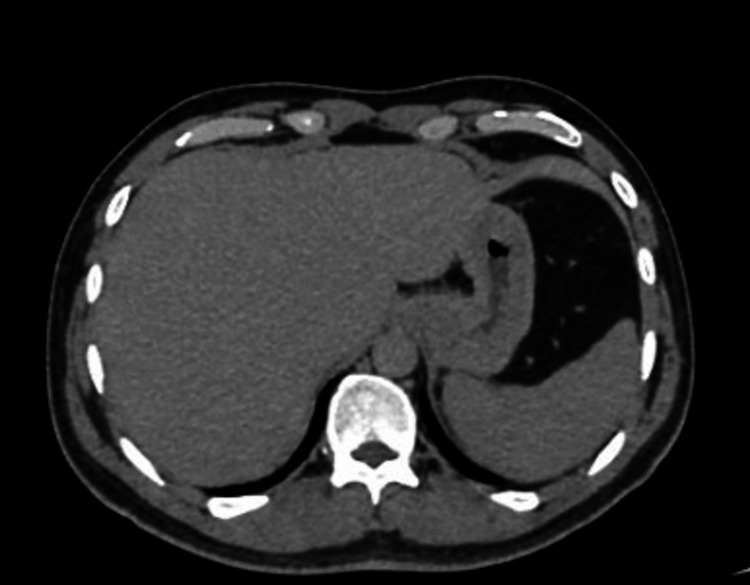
Normal abdominal CT scan CT: computed tomography
